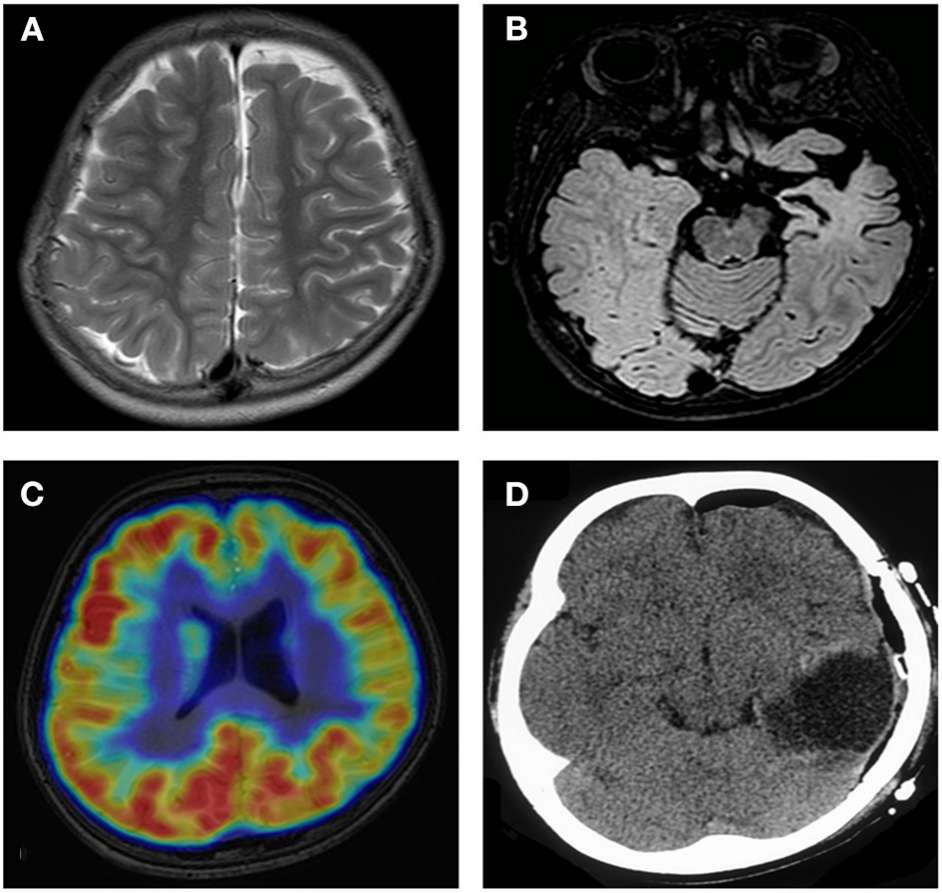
Neuroimaging data of case 4. MRI shows mild atrophy in the left hemisphere (A). MRI reveals encephalomalacia in the left temporal lobe (B). PET shows hypometabolism in the frontal and parietal lobes (C). Imaging shows completed left temporal lobectomy (D).
